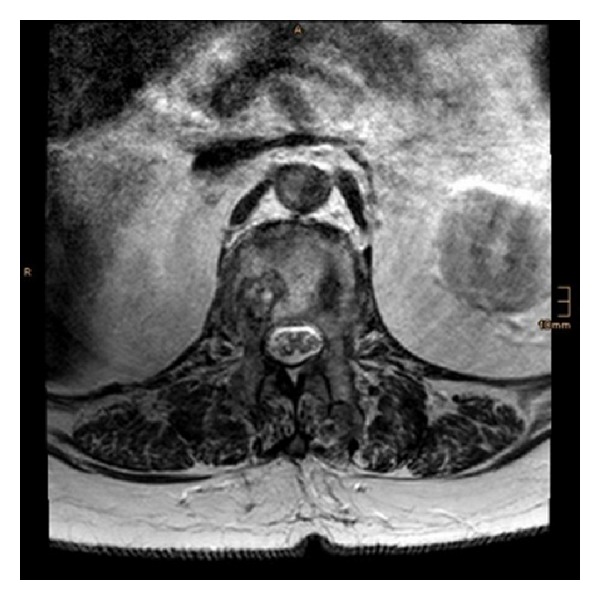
Postoperative axial MRI (T2-weighted) at the level of L1.
